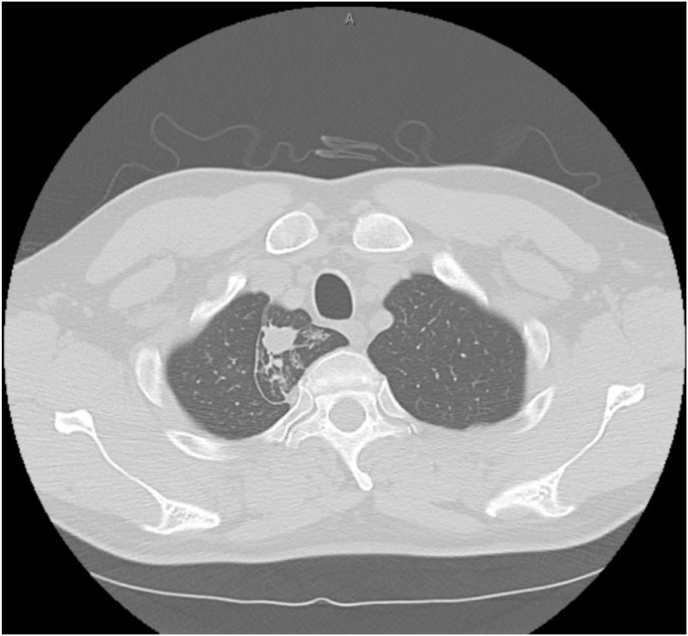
A computed tomography (CT) scan of the chest showing multiple nodules, including in the azygous lobe, right upper lobe, and right lower lobe, with associated micronodular patterns.
